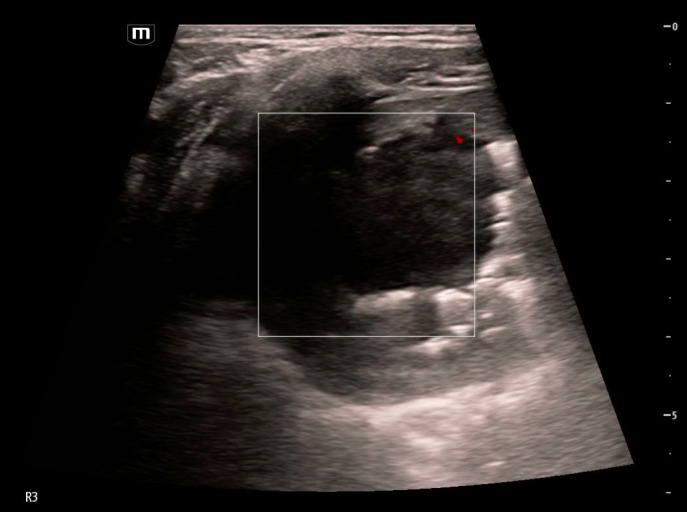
Cavitary lesion with color doppler demonstrating no vascular flow within the cavity.
